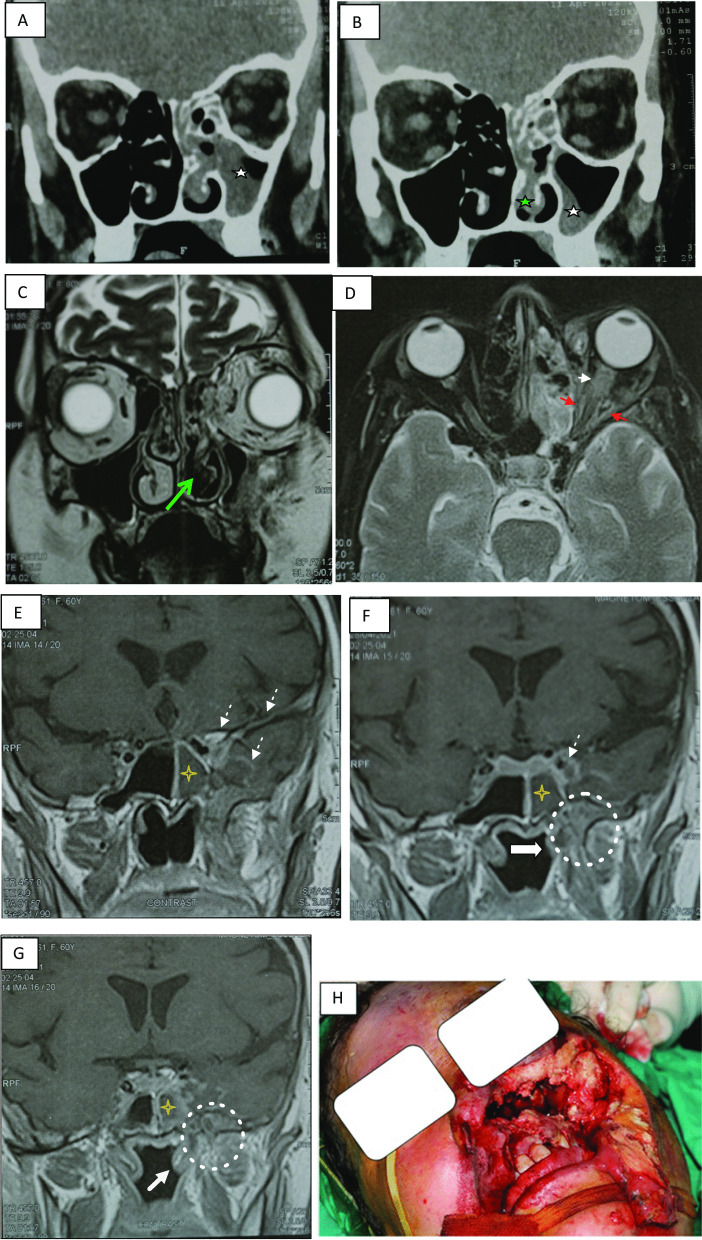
A 60-year-old female patient presented with left proptosis, visual loss, total ophthalmoplegia and left facial pain after 3 weeks of COVID-19 infection with poor diabetic control. A, B CT coronal images show mucosal thickening of the left maxillary sinus (white asterisk) and left nasal cavity (green asterisks). C MRI coronal T2WI shows stranding in the orbit fat, involvement of left nasal cavity (black turbinate sign). D MRI axial contrast-enhanced T2WI with fat suppression image shows non-enhancement and thickening of left optic nerve (white arrowhead), hypointense soft tissue intensity in left orbital apex (red arrowheads) with orbital fat stranding. E–G Coronal contrast-enhanced T1WI shows left leptomeningeal thickening and enhancement along the floor of the middle cranial fossa and abnormal enhancement in the left temporal lobe, left cavernous sinus (dashed arrows). Meckel’s cave is infiltrated with extension along the left mandibular nerve (white dashed circle) to infratemporal fossa, mucosal thickening of the left sphenoid sinus (yellow asterisks), and nasopharynx (thick arrow). H Operative view shows necrosis of the facial soft tissue and sinus. Patient underwent aggressive debridement in the form of left total maxillectomy and left orbit exenteration, patient survived after proper diabetic control and IV amphotericin B administration
